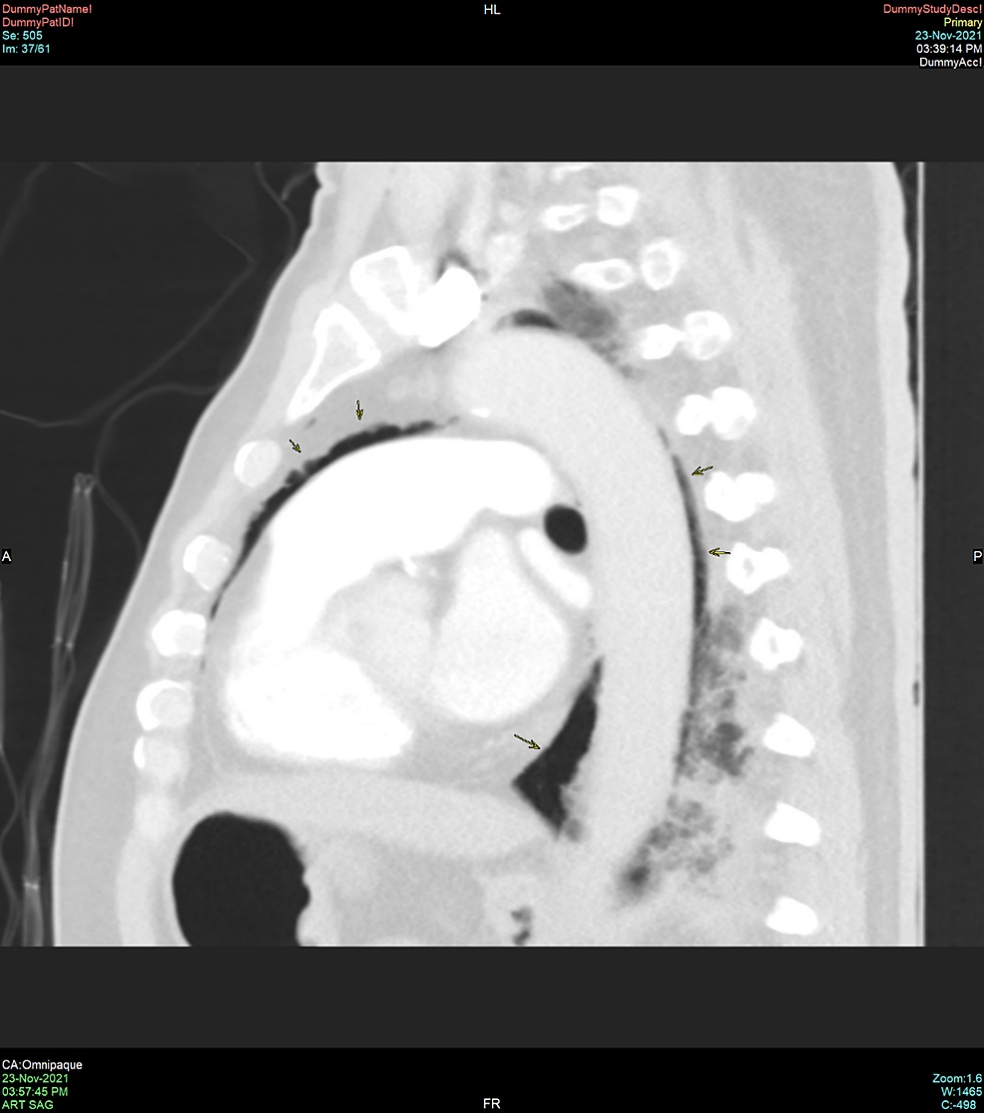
Sagittal view of a computed tomography scan of the thorax demonstrating pneumomediastinum (black arrows)
